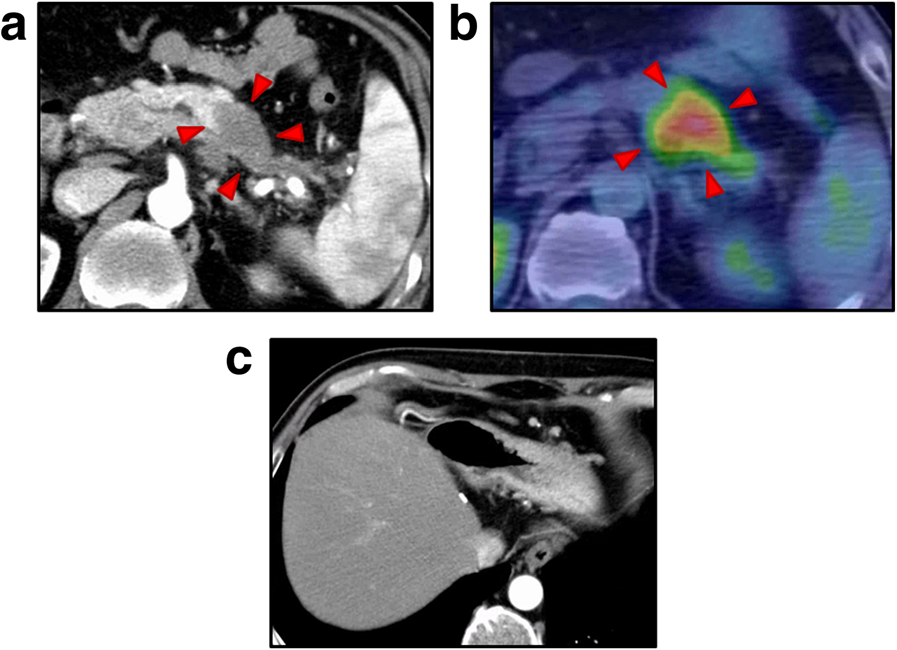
Preoperative imaging of the primary pancreatic cancer. Enhanced computed tomography (CT) showed a 30-mm solid tumor in the tail of the pancreas (a, arrowheads). Positron emission tomography–CT showed abnormal accumulation in the tumor (b, arrowheads). There was no gastric before the initial surgery (c)
